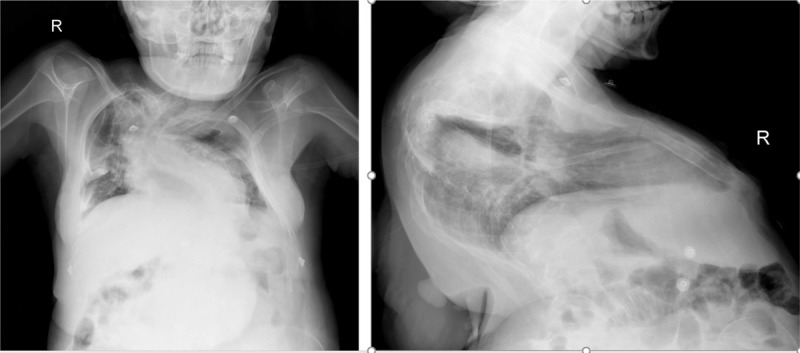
X radiographs of chest imaging at the position of coronal and oblique-axial plane.
